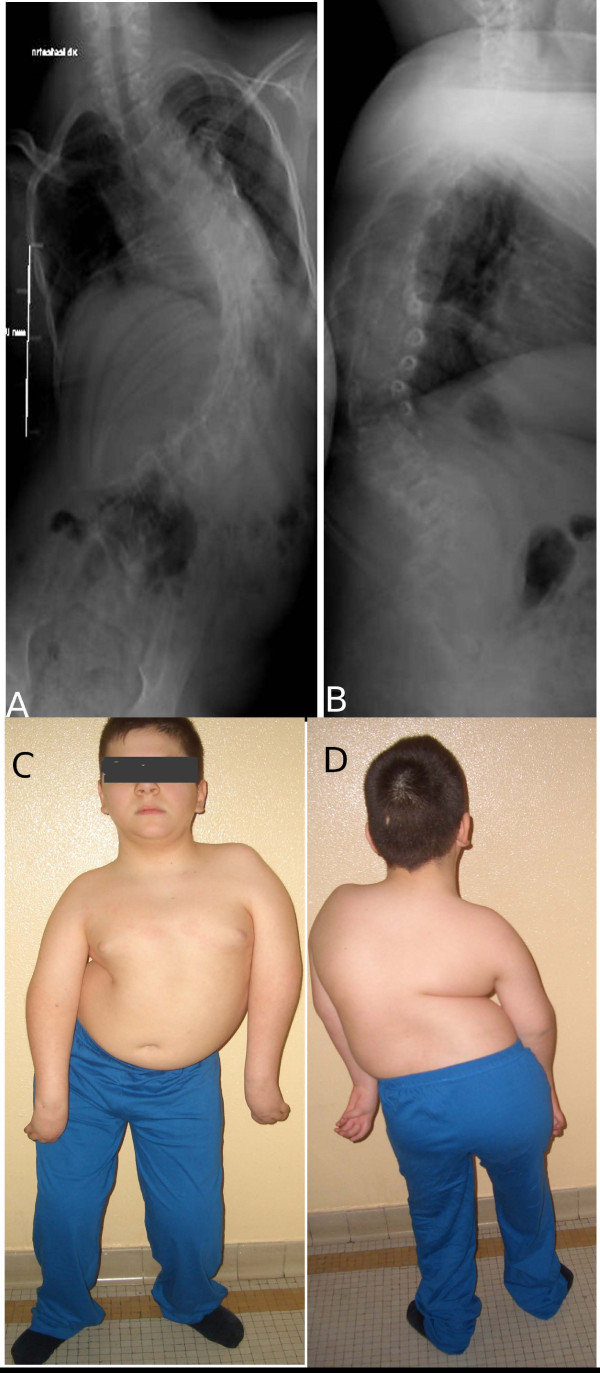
(A, B, C, D), Case 5: preoperative, radiographic and clinical pictures.
